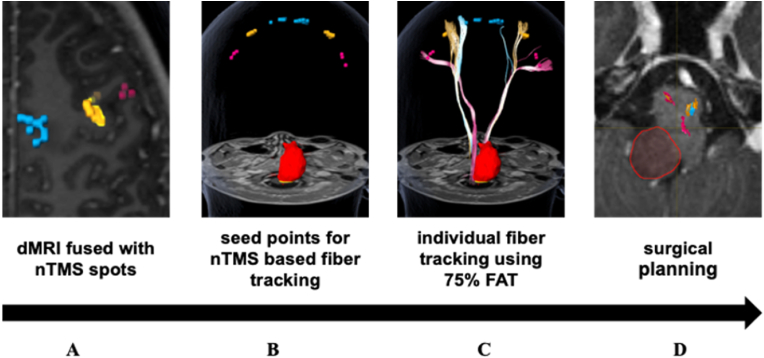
Preoperative protocol. The preoperative protocol starts with the MRI scan (including diffusion weighted imaging and DTI), followed by the nTMS examination for determination of primary motor area (A&B) and seed points, nTMS based fiber tracking (C&D) and the surgical planning.
